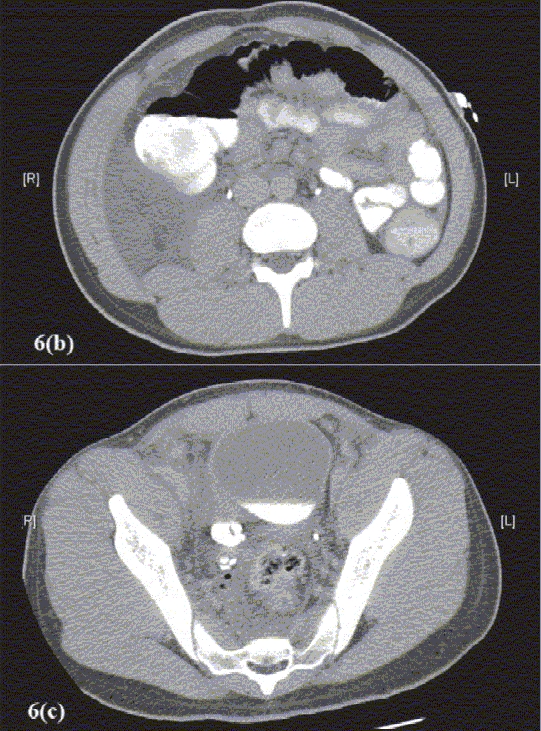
Axial sections of CT scan demonstrating normal ureteral anatomy in the abdomen (b), but the pelvic portion of the right ureter has contrast extravasating at the level of the right UVJ (c)
